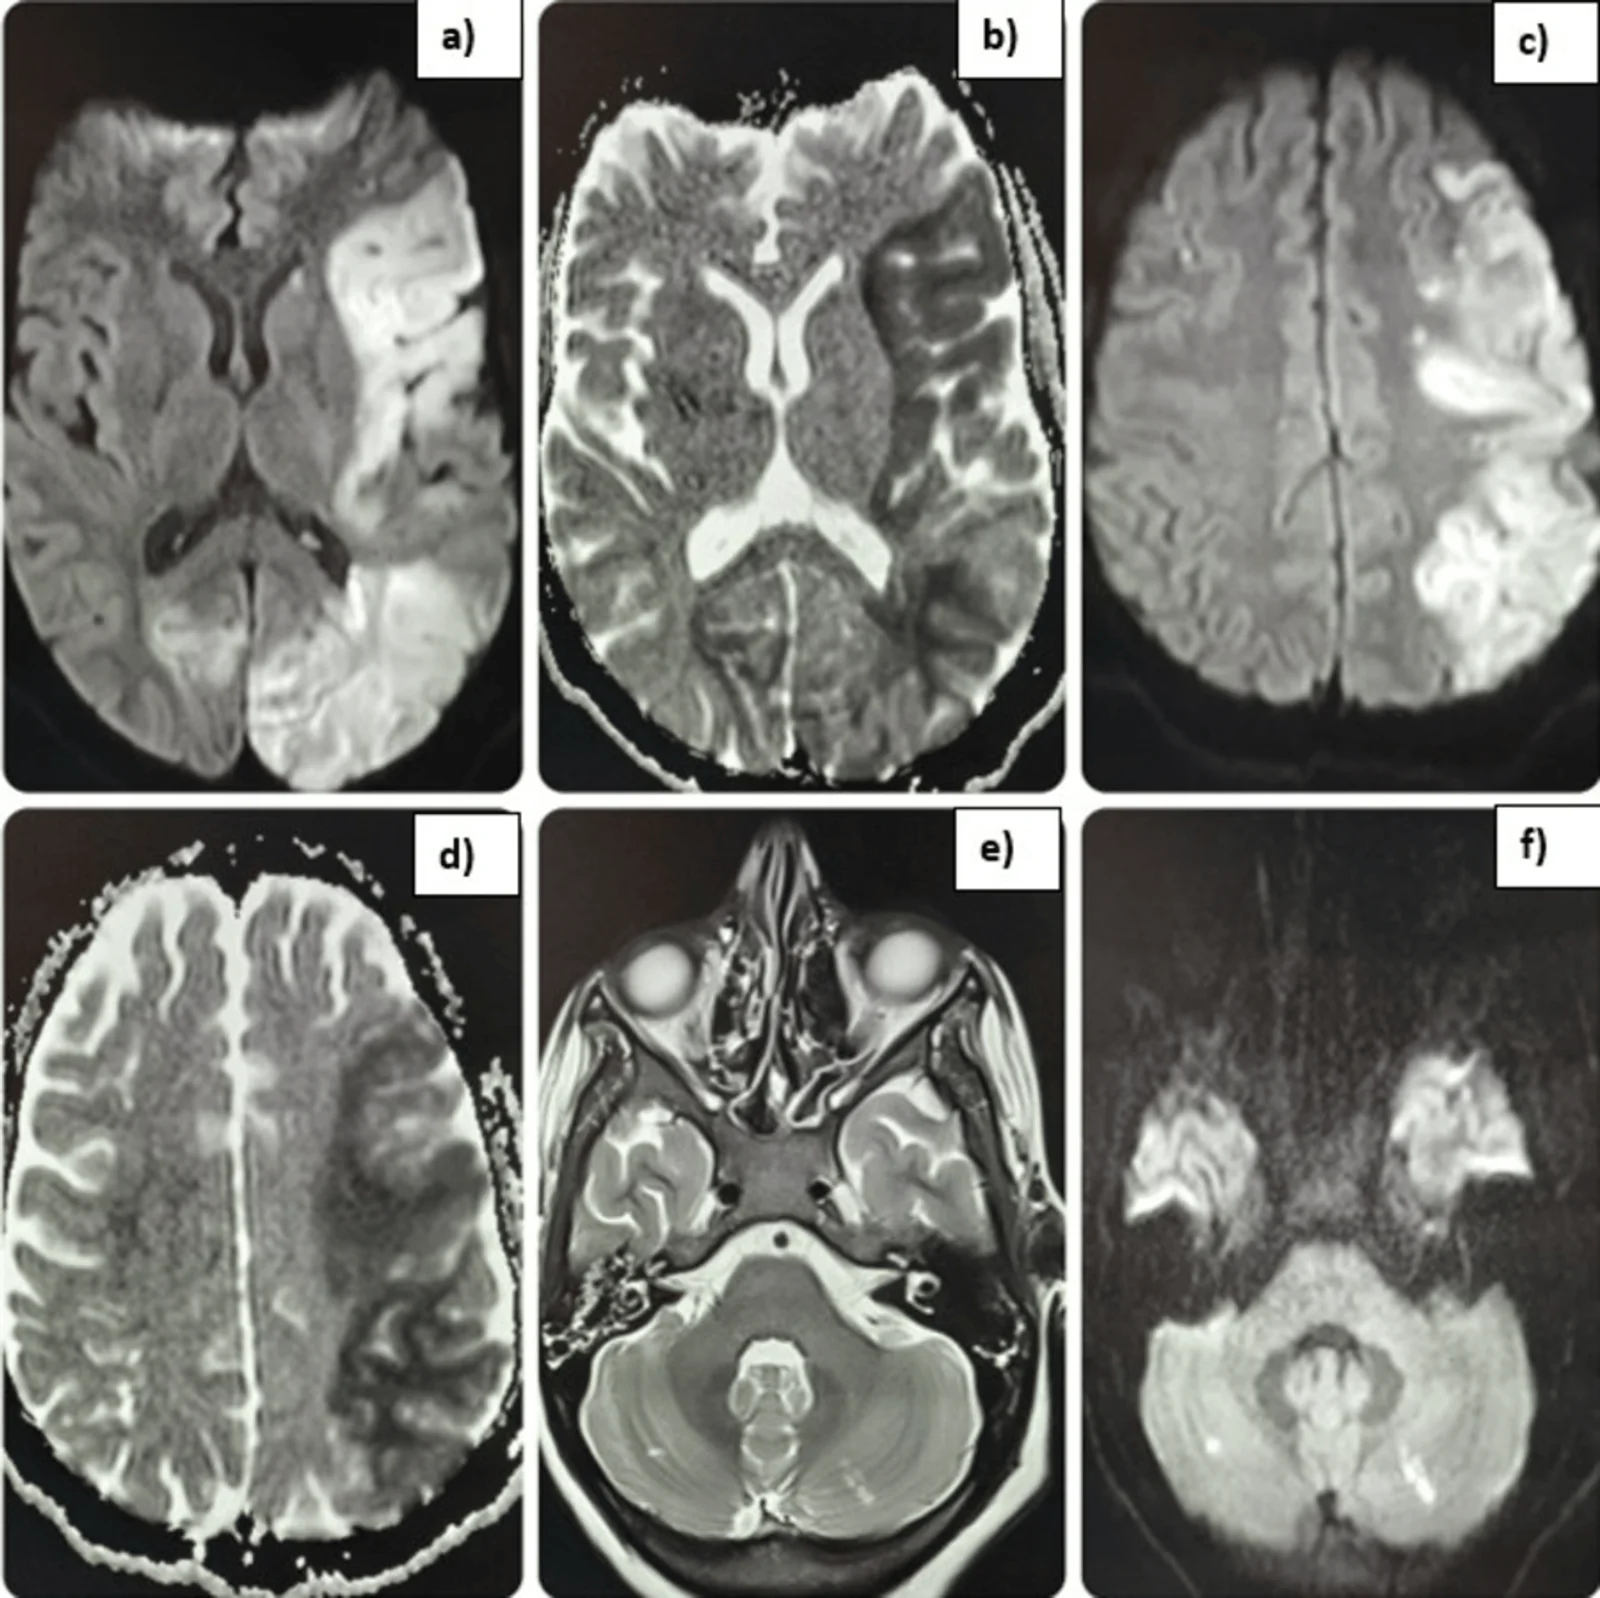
MRI brain demonstrating multifocal acute ischemic infarctions with diffusion restriction. (a) Axial DWI demonstrates a large area of diffusion restriction involving the left parieto-temporo-occipital region and smaller similar areas in the right occipital region, suggestive of acute infarction in the left middle cerebral artery and bilateral posterior cerebral artery territories. (b) Corresponding ADC map shows low signal intensity in the same region, confirming restricted diffusion consistent with acute infarction. (c) Axial DWI at a higher level demonstrates additional areas of diffusion restriction involving the left parietal and occipital lobes. (d) Corresponding ADC map confirms restricted diffusion in the involved cerebral regions. (e) Axial T2-weighted image demonstrates hyperintense signal changes involving bilateral cerebellar hemispheres. (f) Axial DWI demonstrates bilateral cerebellar diffusion restriction, consistent with acute infarcts in bilateral posterior inferior cerebellar artery territories MRI: magnetic resonance imaging; DWI: diffusion-weighted imaging; ADC: apparent diffusion coefficient
